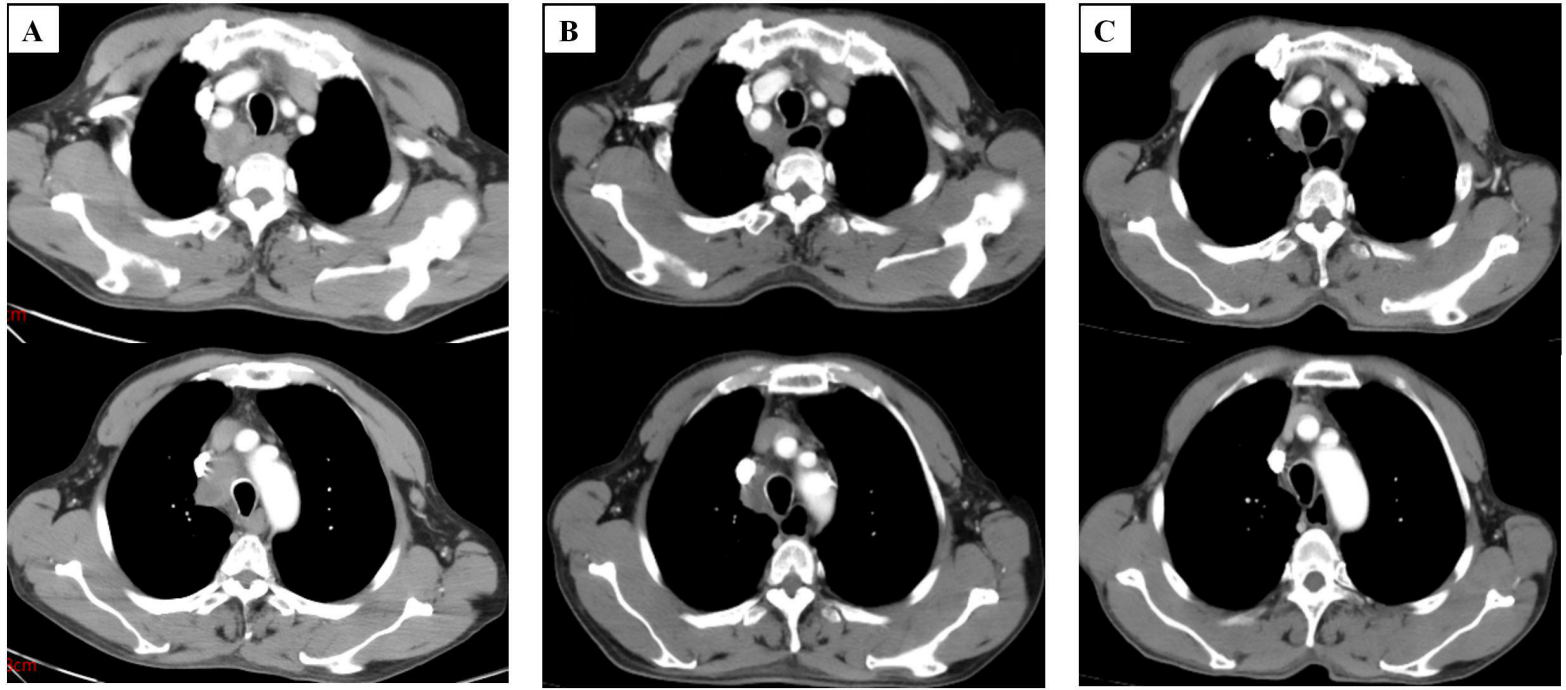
Computed tomography images of the patient. (A) Computed tomography image shows enlarged lymph nodes in the mediastinum before chemotherapy (January 04, 2024). (B) Computed tomography images of patients after 2 cycles of chemotherapy(February 23, 2024). (C) Computed tomography images of patients after 4 cycles of chemotherapy (April 8, 2024) (slice thickness: 5 mm; window width: 360; window level: 60; arterial phase).
